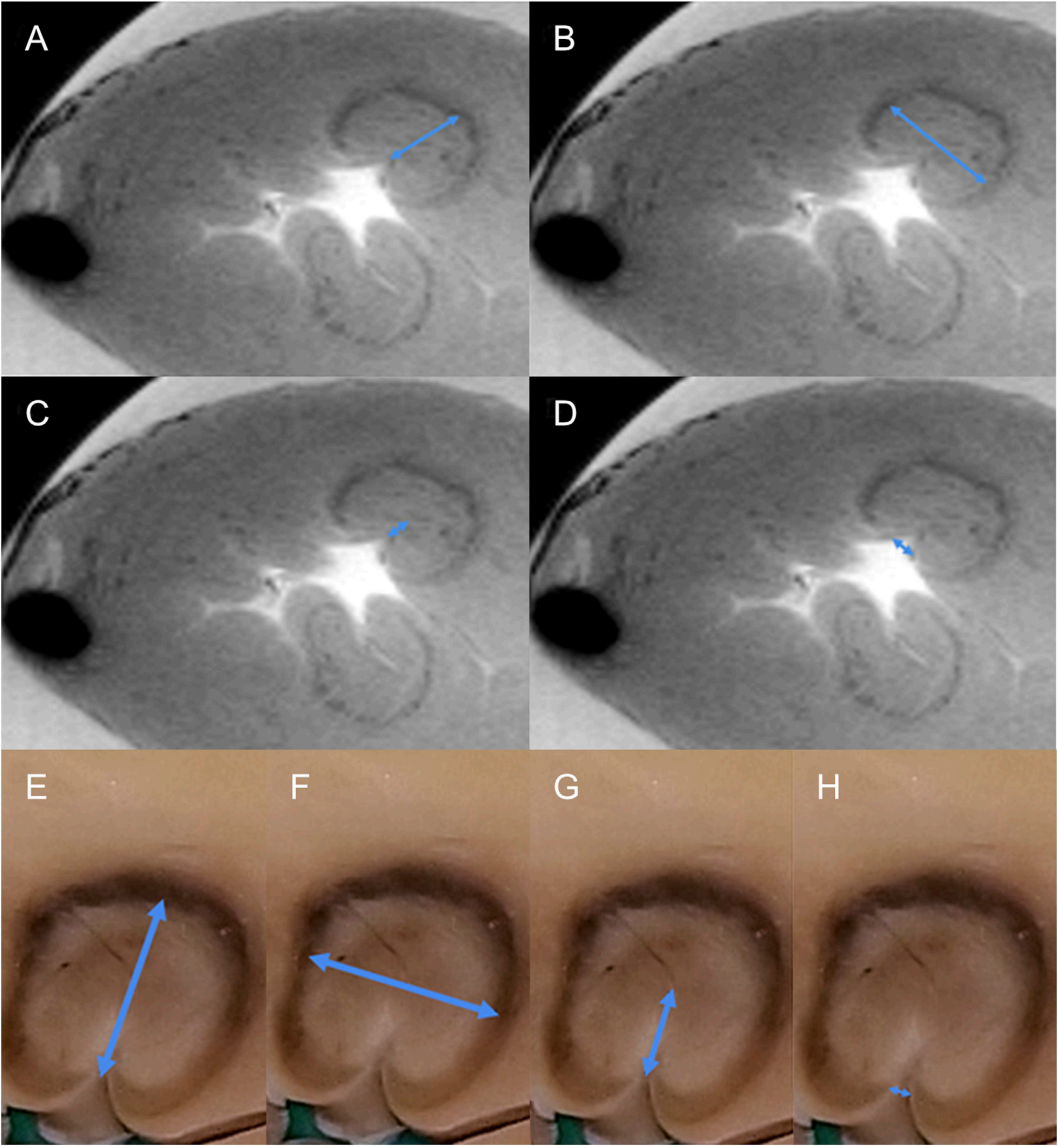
Example of measurement of the different dimensions of the radiofrequency lesion. (A–D), measurement from MRI data, transverse section of the left ventricle. Two lesions are visible, the upper one is measured (blue arrows, A = depth, B = width, C = depth at the maximum diameter, and D = lesion surface diameter (“estuary). (E–H), measurement from pathological samples, detail of the lesion, the same measurement as for MRI [blue arrows, E = depth, F = width, G = depth at the maximum diameter, and H = lesion surface diameter (“estuary”)].
